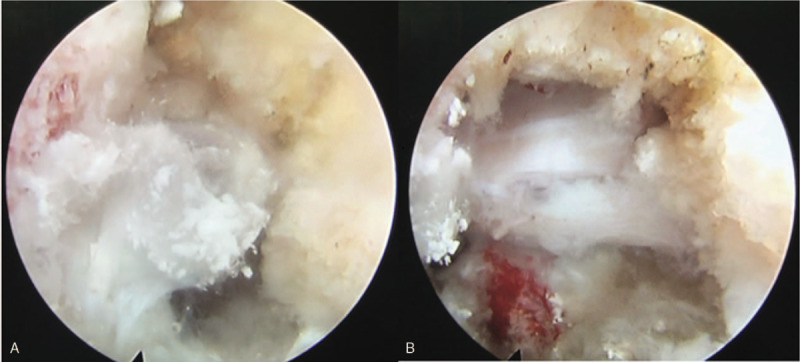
Intraoperative view (A) showed the chalky white material was occupying the epidural space and infiltrating the soft tissue. (B) After removing most of the chalky white material and the extruded nucleus, the transverse nerve was fully decompressed and could move with the heart rate and cough. CT = computed tomography, MRI = magnetic resonance imaging.
